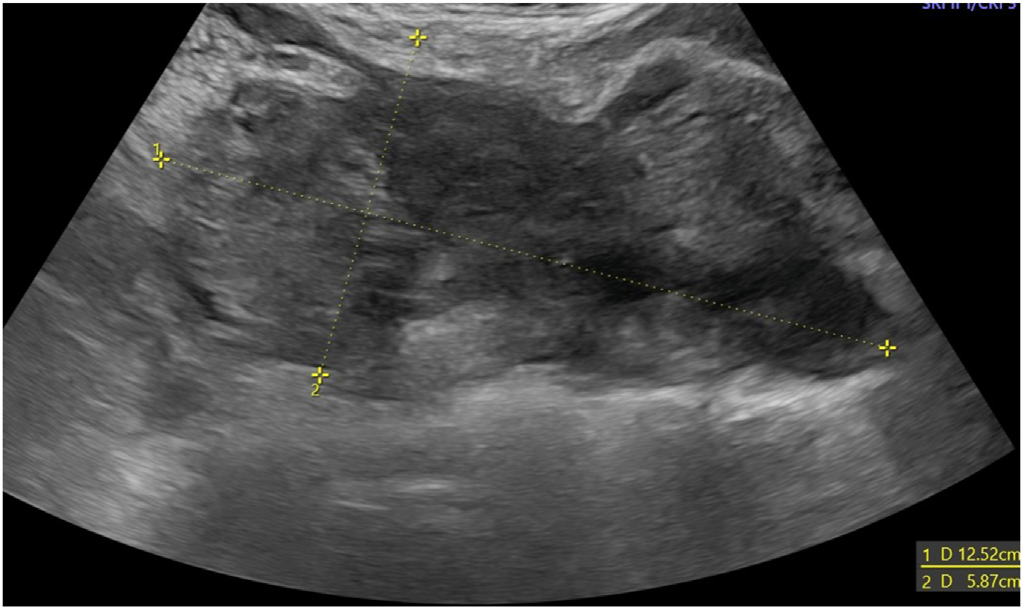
With transabdominal ultrasound, a large nonhomogeneous mass is seen in the right adnexa. Reprinted from content created by Dr. Salma Babikir Ahmed Erabi from Radiopaedia.org, rID:83742, under CC BY-NC-SA 3.0 license (https://creativecommons.org/licenses/by-nc-sa/3.0/).
